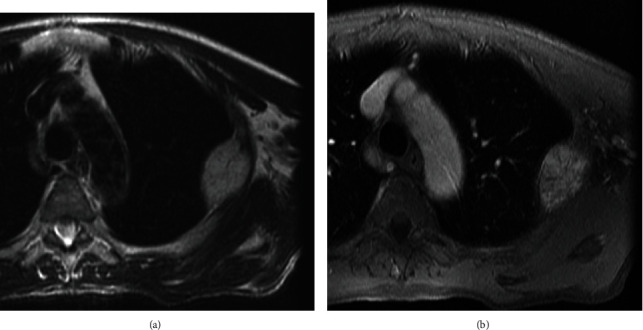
Magnetic resonance imaging showing the tumor in the 4th rib. (a) The mass was hyperintense on T2-weighted images. (b) The mass showed enhancement on gadolinium-enhanced, fat-saturation T1-weighted images.
